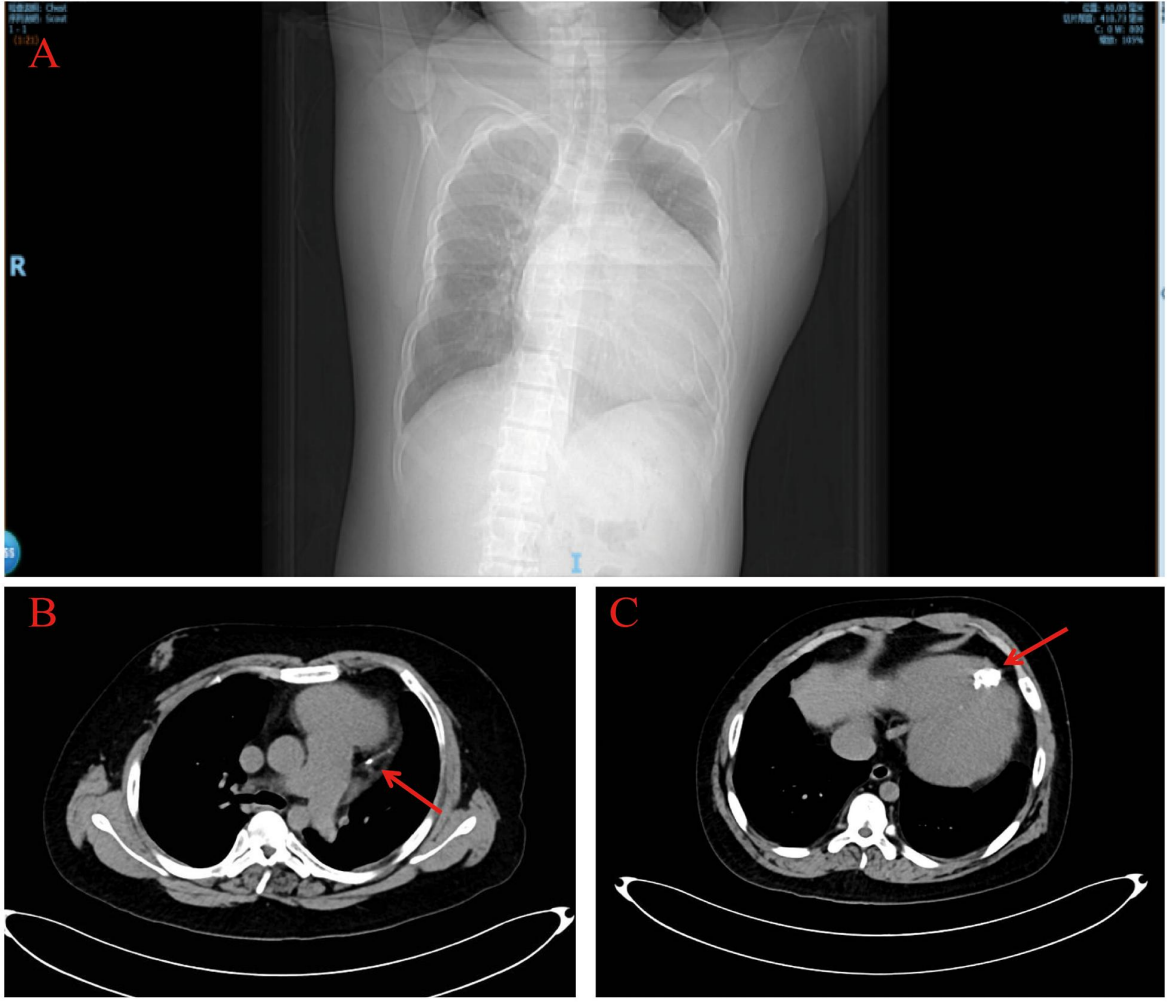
Chest computed tomography. The chest computed tomography revealed a significantly enlarged, spherical heart (A) and calcifications at the cardiac apex (red arrows) (B,C).
